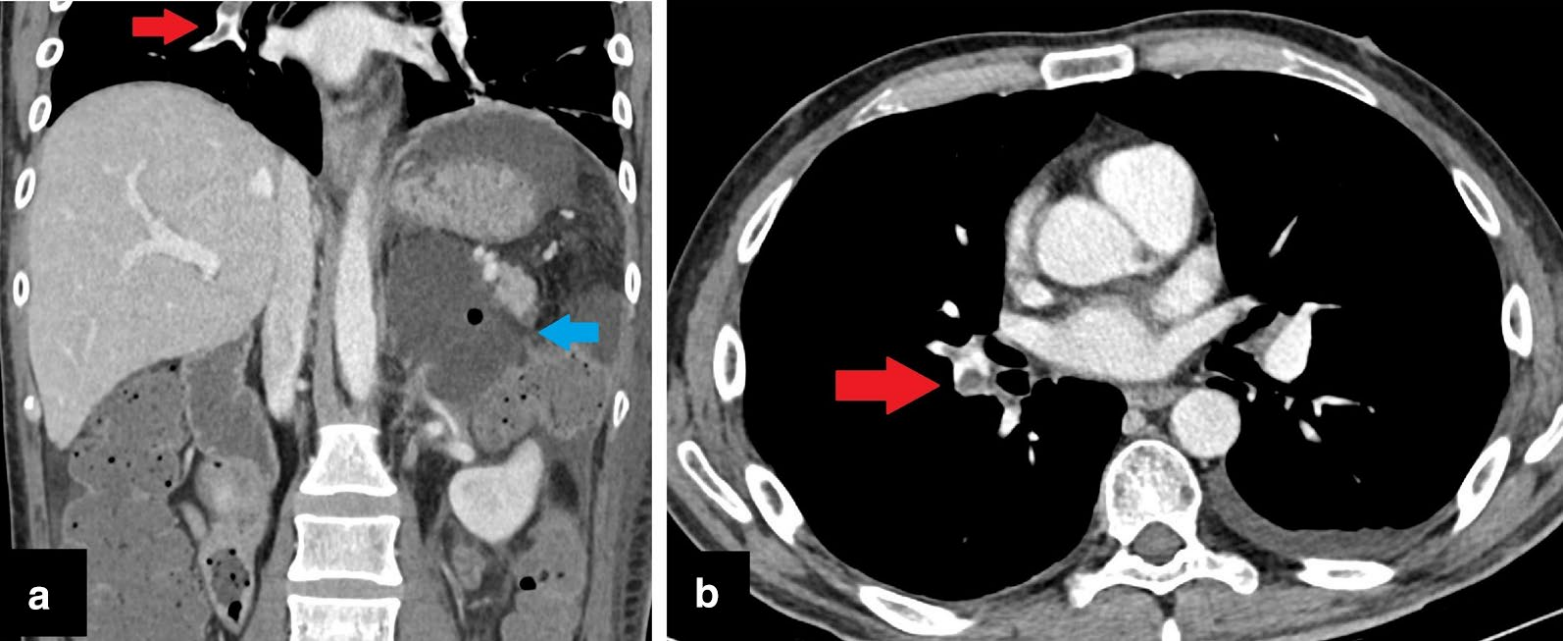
Location-related (type 9) error with under-reading. In a 28-year-old male patient with adrenalectomy, abdominal CECT was performed for evaluation of postoperative complications. Collection in the surgical area (blue arrow, a) and postoperative changes were reported. Although filling defects in the right pulmonary artery were involved in upper CT slices, they could not be noticed due to their locations (red arrows, a, b). As in this case, the marginal areas are prone to this type of error. There is also an accompanying under-reading error because the pulmonary emboli could be clearly seen and should have been reported
